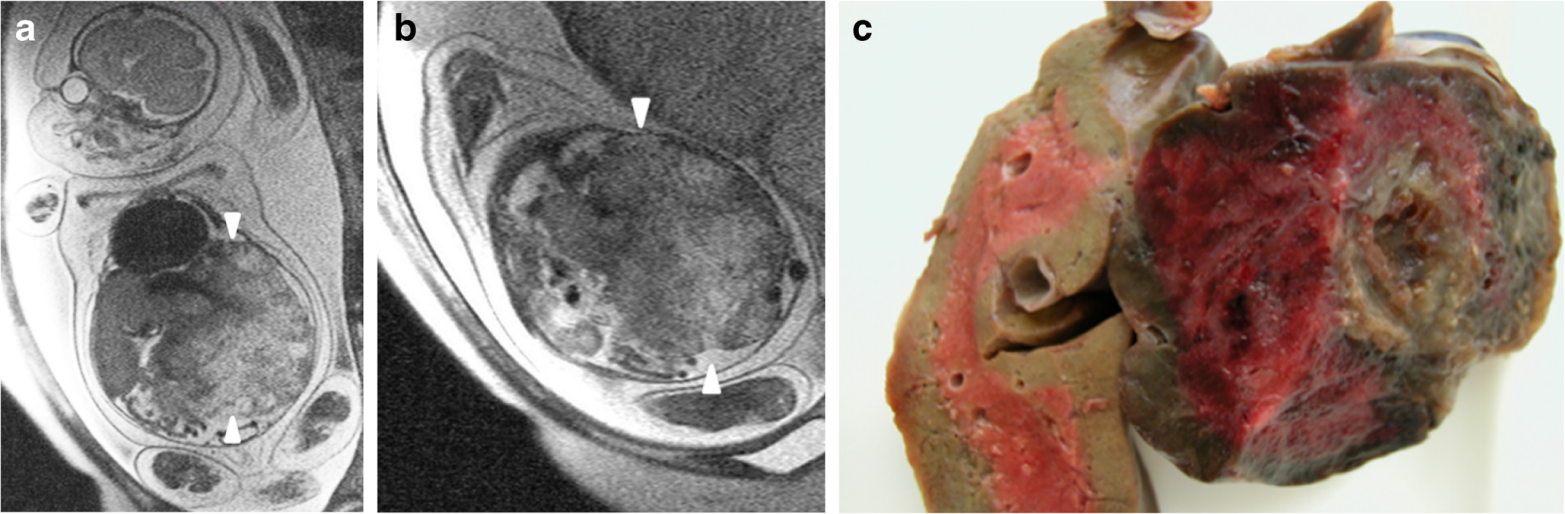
A male fetus at 27 weeks’ gestation with hepatic hemangioma (N23). a–b Coronal (a) and left sagittal (b) T2-weighted MR images (repetition time/echo time 1,530/86 ms) show a huge, heterogeneous left abdominal mass arising from the left hepatic lobe. Note the absence of tubular intralesional structures. *Arrowheads* show the borders of the lesion. The tumor causes bilateral elevation of the hemidiaphragms and severe secondary lung hypoplasia, fetal cardiomegaly and marked hydrops fetalis. The patient died at birth after an emergency cesarean at the 27th week of pregnancy. c Macroscopic view of the fetal liver confirms the hepatic origin of this tumor and reveals central cavities and extensive necrosis
